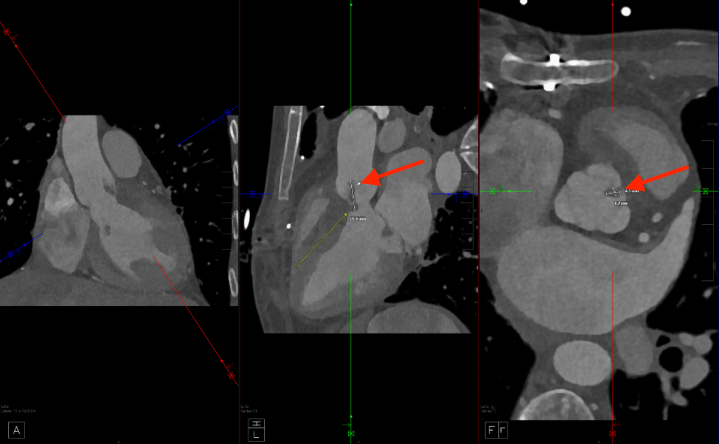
Aortic Valve Lesion Extending Into Left Ventricular Outflow Tract on Cardiac Computed Tomography Cardiac computed tomography showing filling defect attached to right aortic valve leaflet extending into the left ventricular outflow tract (arrow).
